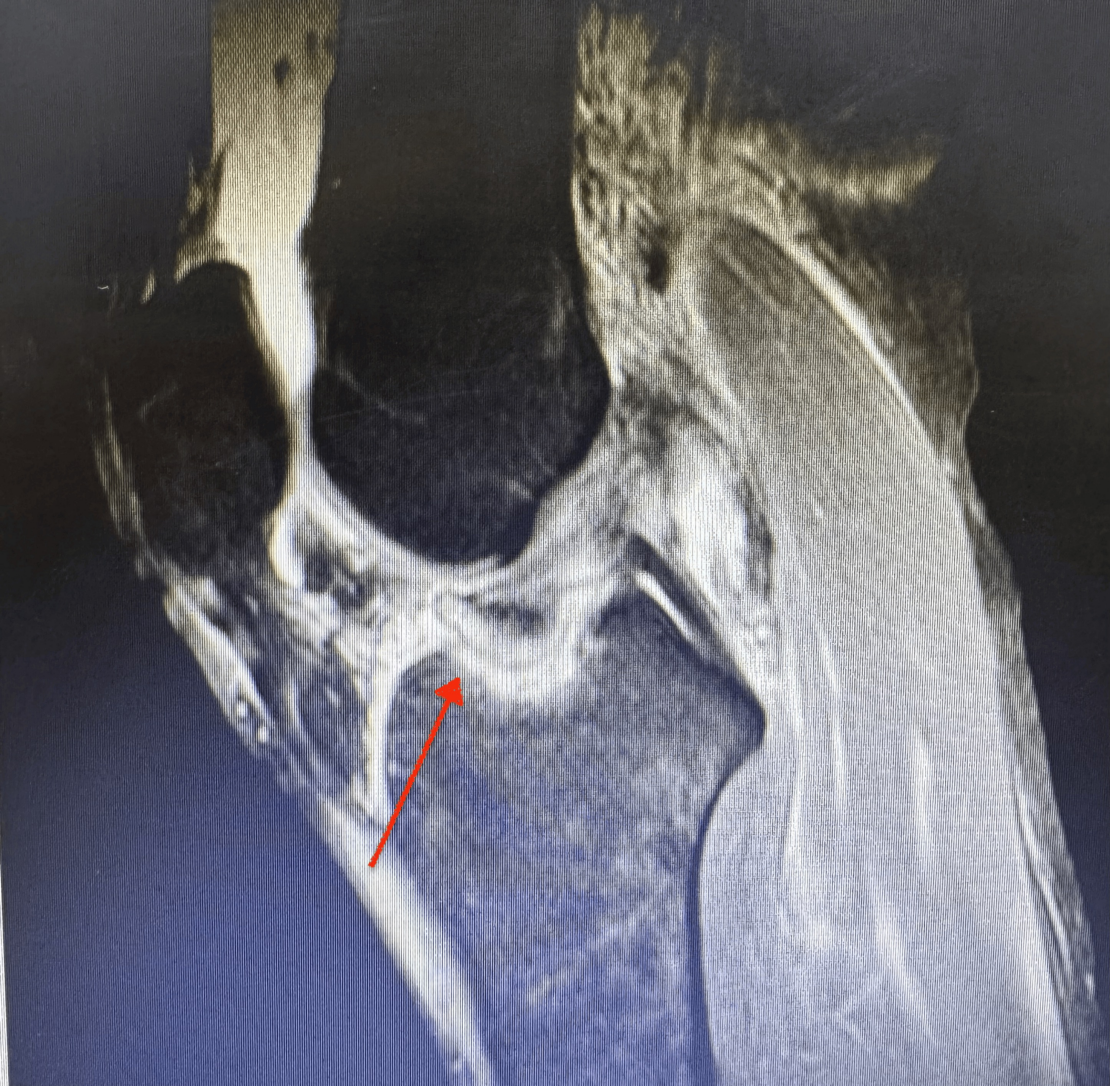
MRI sagittal view of the left knee demonstrating the site of the tibial spine avulsion fracture (red arrow)
